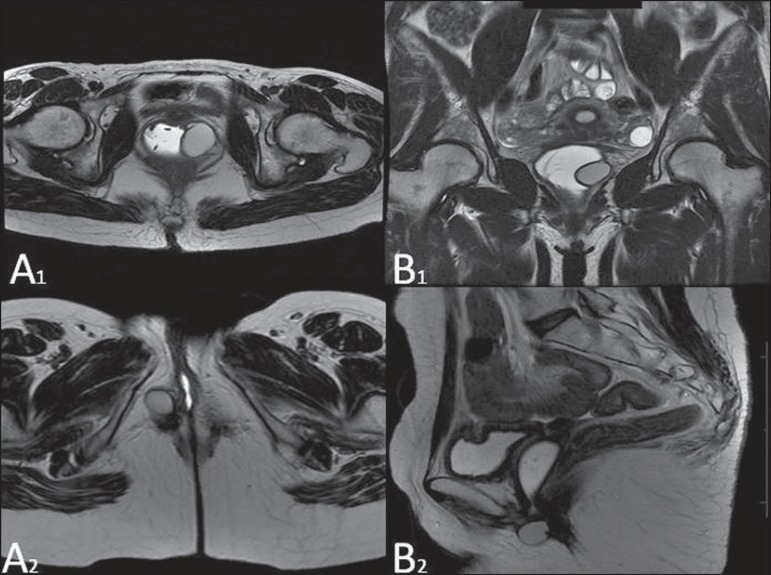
Gartner duct cyst – axial (A1) and sagittal (B1) MRI
